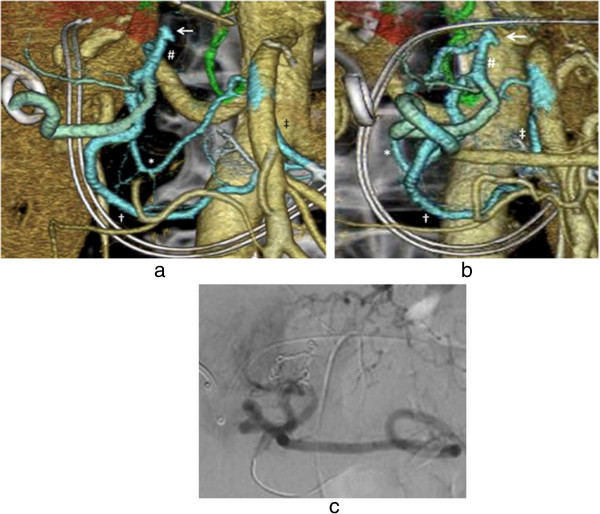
A case of bleeding post-pancreatectomy following Appleby operation for pancreatic body cancer with ligation of the common hepatic artery. A hemorrhage-responsible arteriogram created by CT during abdominal aortography depicts the pseudoaneurysm (arrow) situated at the end of the gastroduodenal artery (#) on the anterior–posterior view (a); the right anterior oblique view (b) separates the overlapping vessels, enabling microcoil embolization from the posterior superior pancreaticoduodenal artery (*) to the gastroduodenal artery (#) via the anterior superior pancreaticoduodenal (†) arcade, through the anterior inferior pancreaticoduodenal artery (‡) branching from the superior mesenteric artery. Based on the right anterior oblique view, microcoil embolization was conducted (c).
